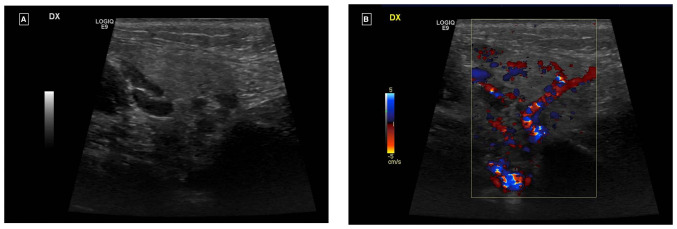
(A) Ultrasound and (B) Ecocolor-Doppler examination of the right parotid gland.
